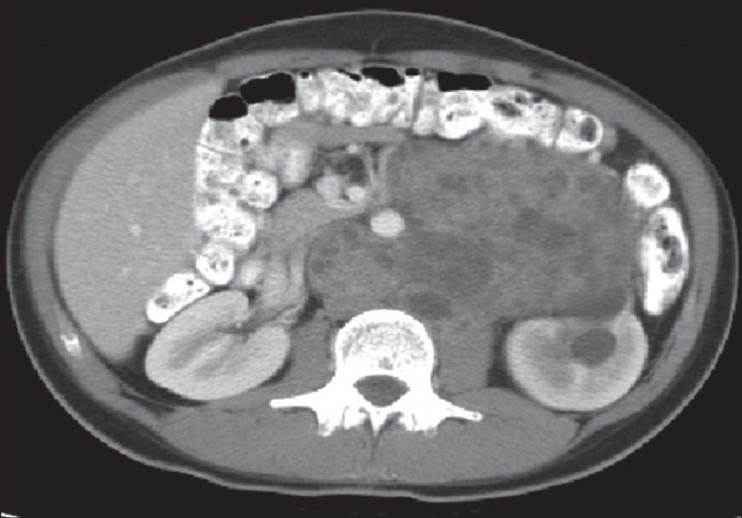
CT scan of patient with normal tumor markers, after chemotherapy
